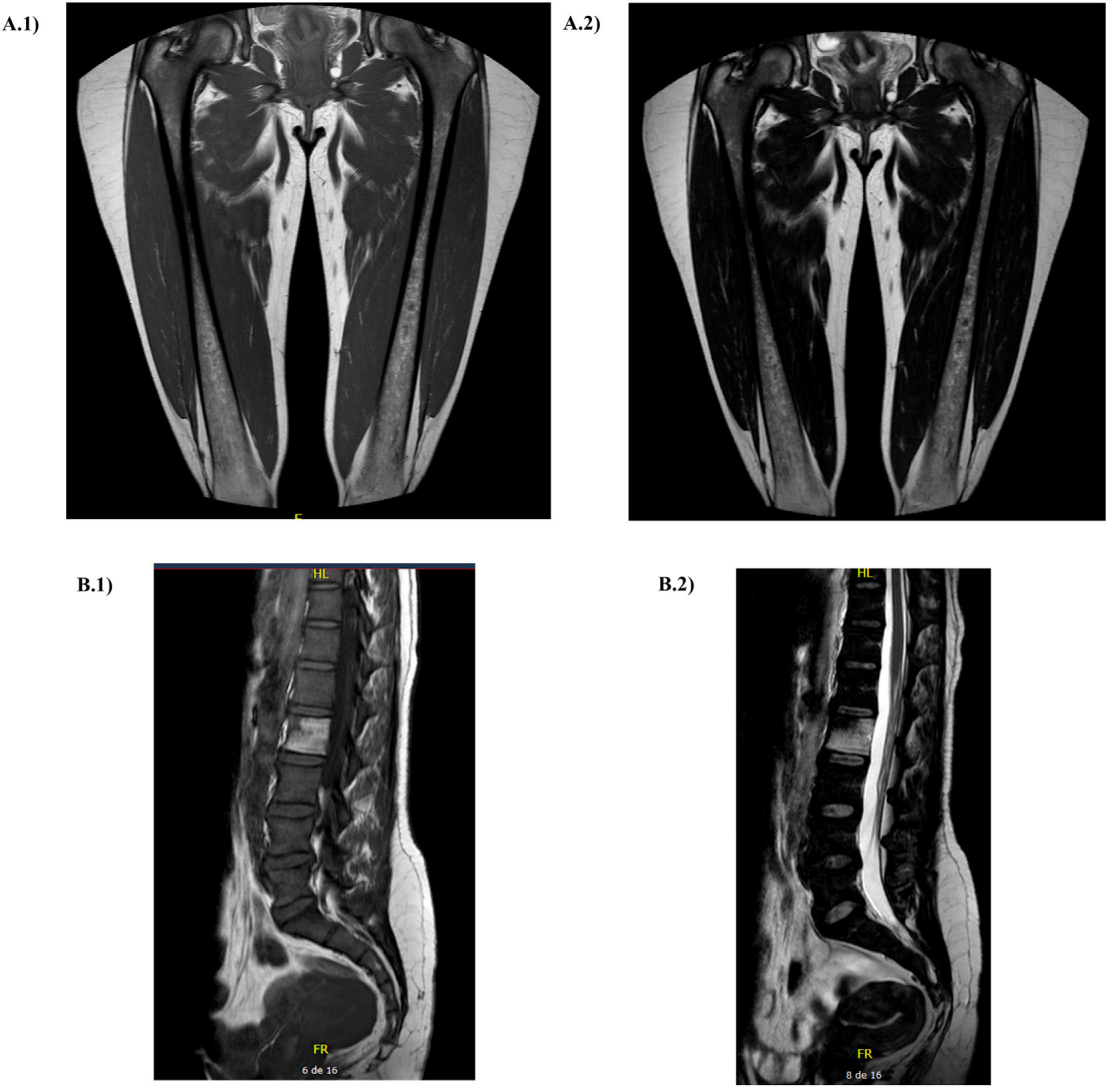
MRI images of patient 1 at baseline. A.1) Coronal T1-weighted image of femurs. A.2) Coronal T2-weighted image of femurs. B.1) Sagittal T1-weighted image of lumbar spine. B.2) Sagittal T2-weighted image of lumbar spine. Femur total score = 6 (T1 = 2, T2 = 2, Sites = 2); Lumbar spine total score = 8 (T1 = 3, T2 = 2, Pattern = 3).
